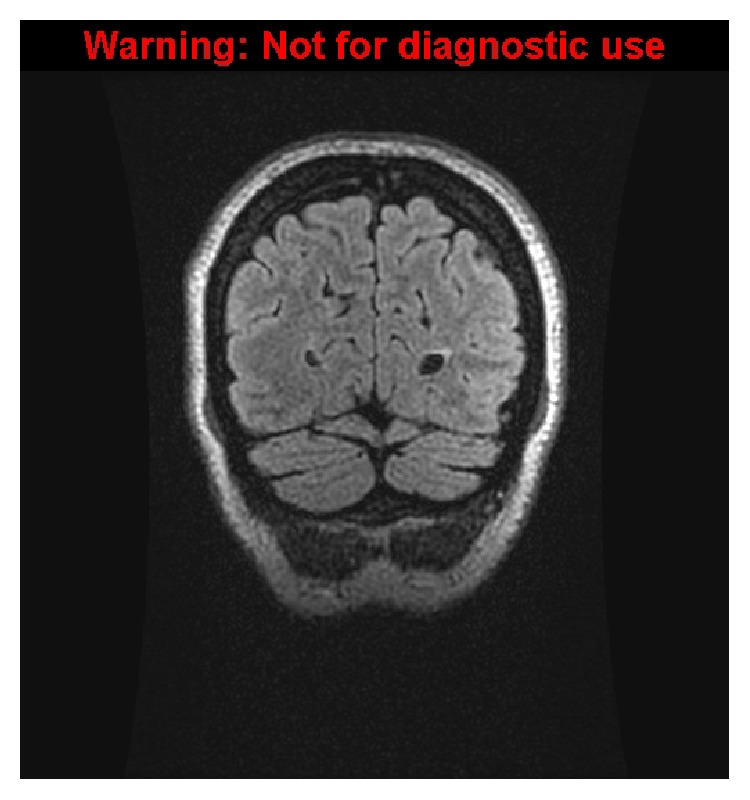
Follow-up coronal FLAIR image one month later demonstrating complete resolution of the hyperintensity noted previously.
